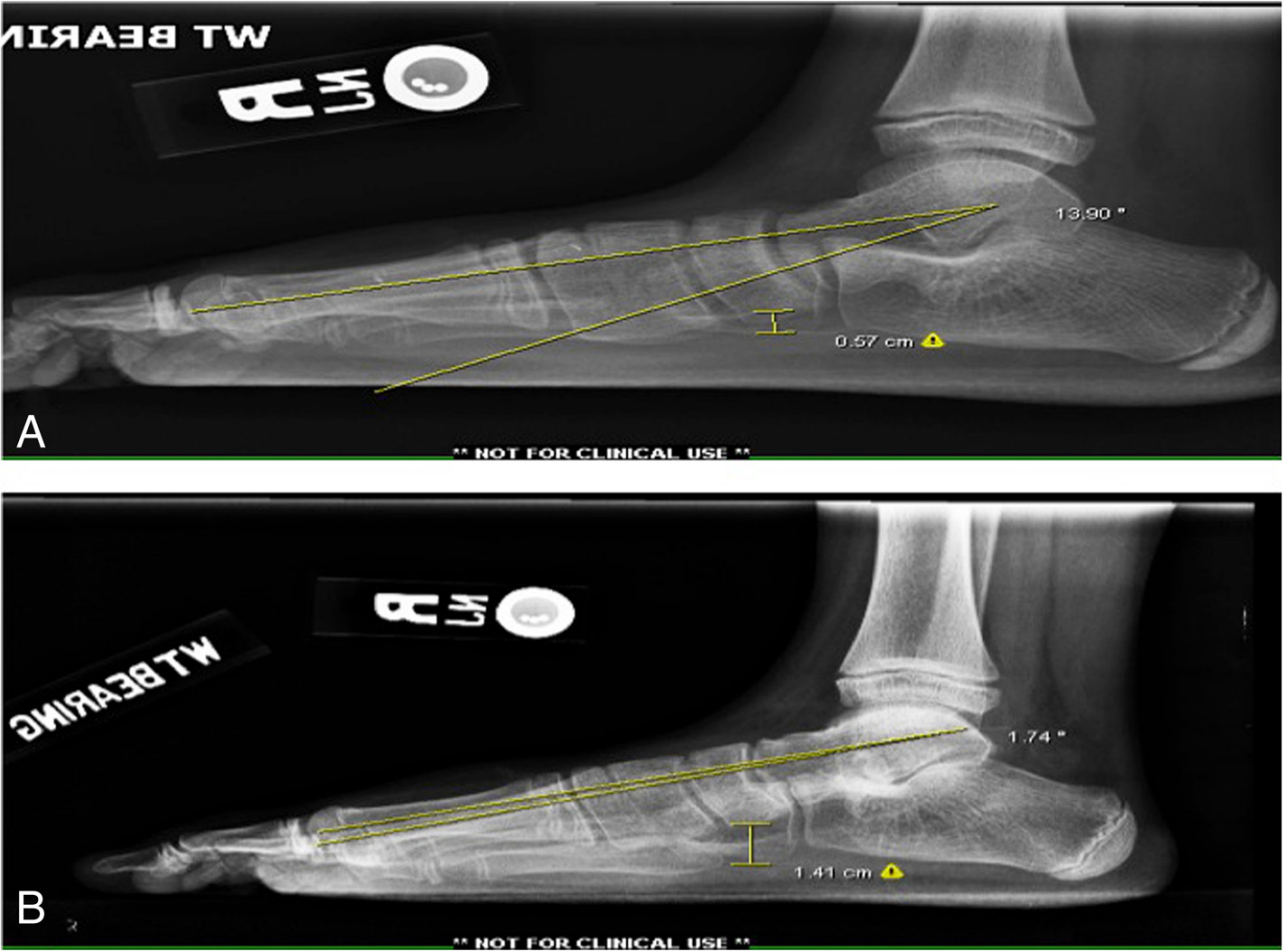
The weight-bearing lateral X-ray shows that the angle of the first tarsometatarsal and the height of the arch in the foot improved (A and B).
